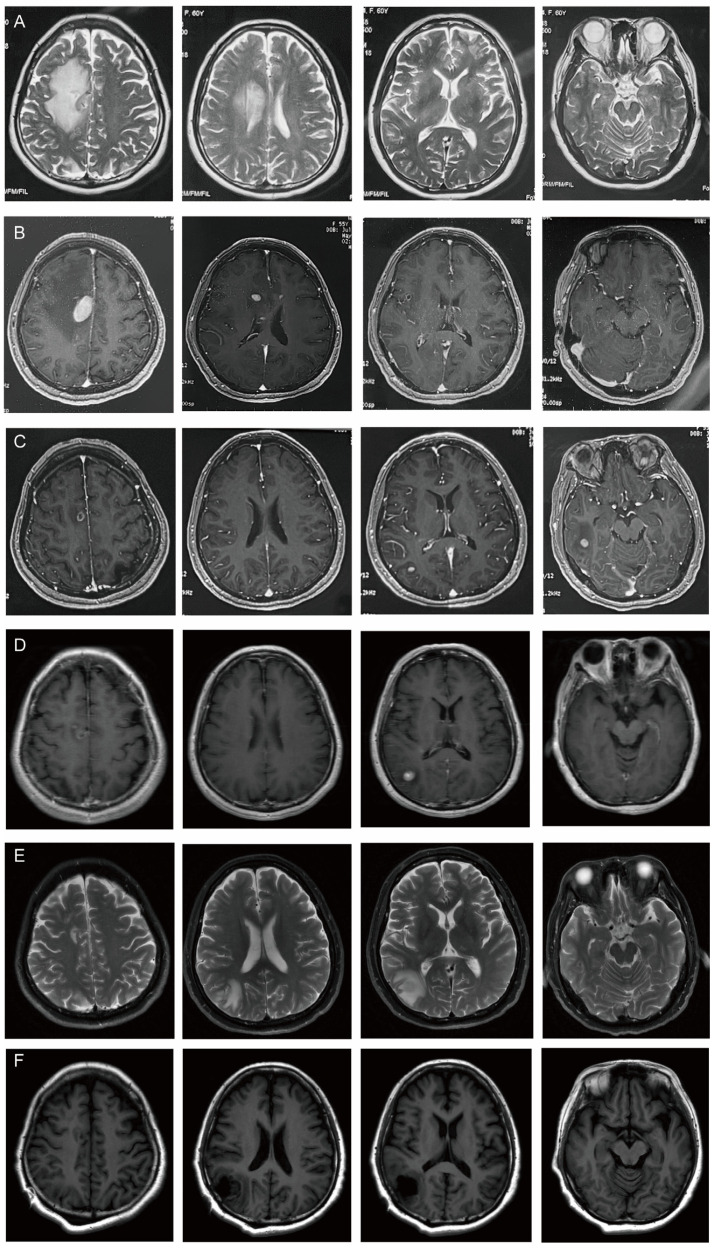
MRI images of patient’s brain during the diagnosis and treatment. Lesions in different parts are shown from left to right: the right centrum semiovale, the top of the lateral ventricle, right occipital lobe, right temporal lobe. (A) 18 April; (B) 6 May; (C) 24 June; (D) 9 July; (E) 22 July; (F) 24 July.
